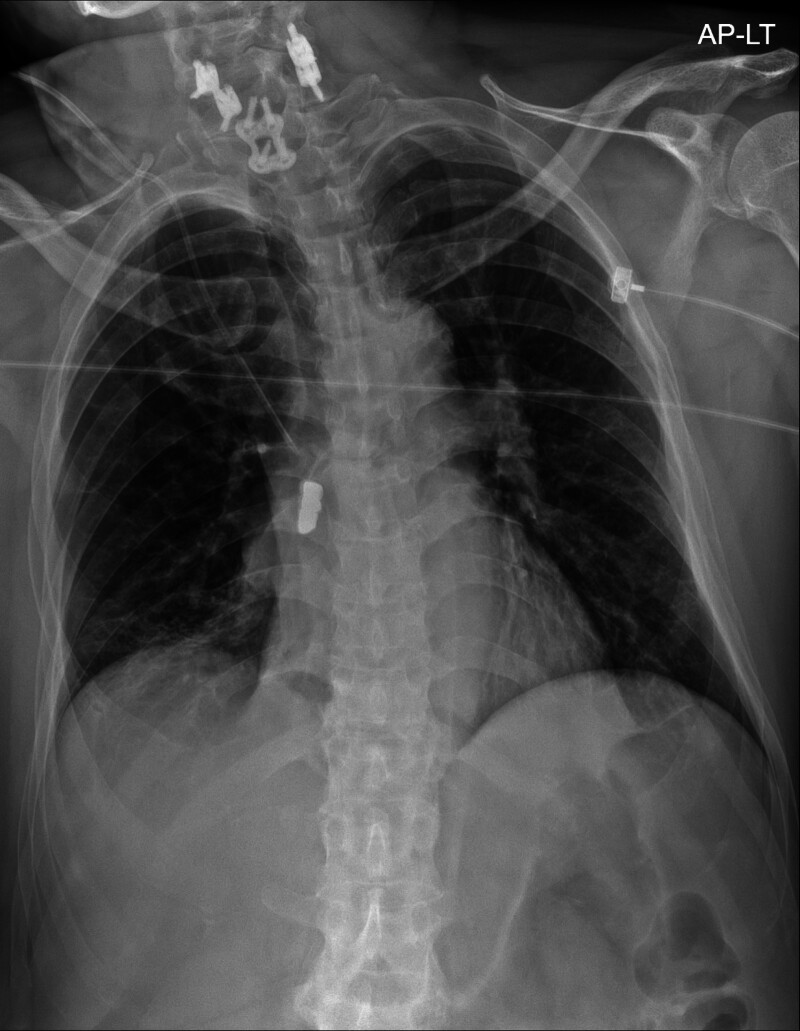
Chest radiography reveals a radiopaque foreign body in the right main bronchus region.
